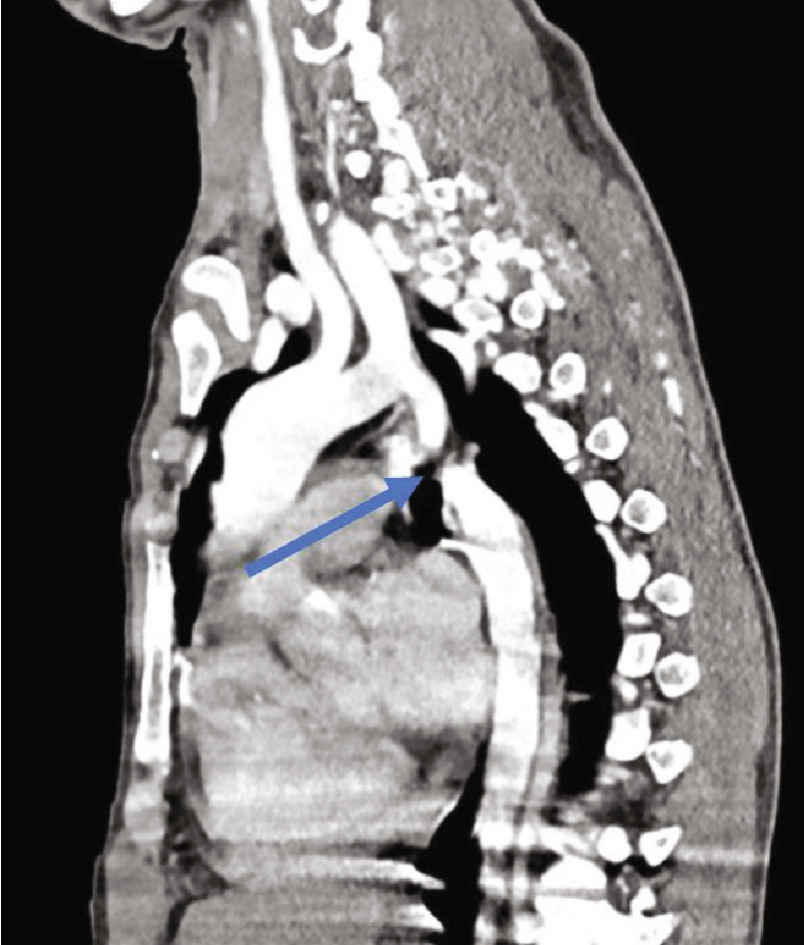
Sagittal reconstructed image of the CT angiography showing a complete discontinuity between the aortic arch and the descending thoracic aorta (arrow).
